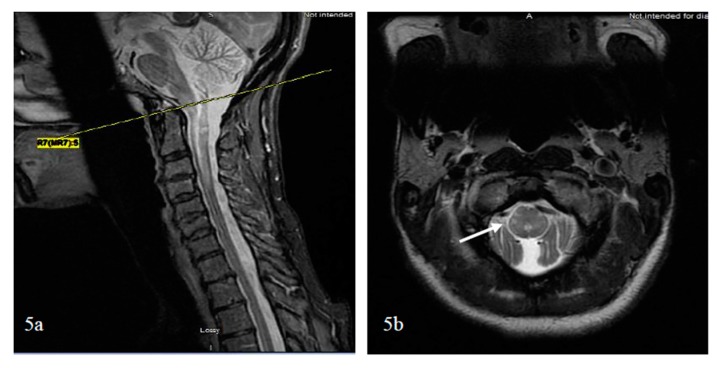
Added to above paragraph 55-year-old African-American female, with AQP4-NMOSD sagittal STIR cervical MRI demonstrates simultaneous linear lesion and LETM from the medulla to C4 (5a). An axial cut at the level of the cervicomedullary junction, axial T2 demonstrates 4 peripheral bright spotty lesions (5b).
